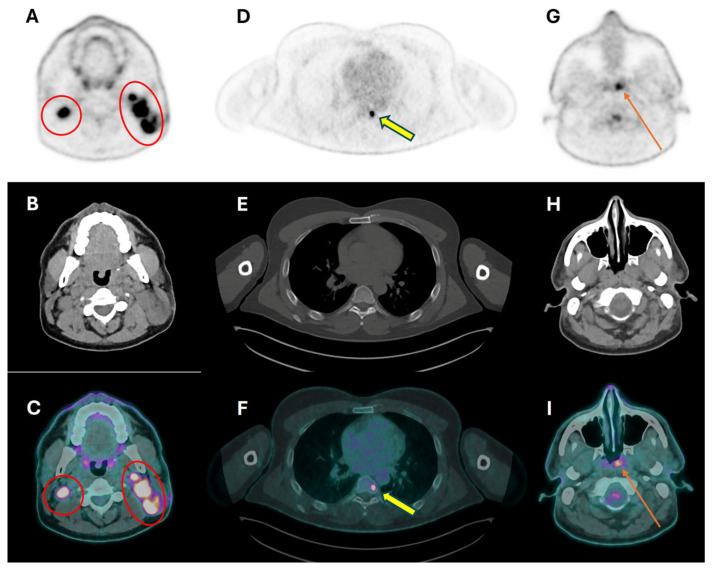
Axial PET, CT, and PET/CT images of a patient with cervical lymph node metastases of undifferentiated carcinoma, and no clinical evidence of primary lesion in the head–neck district. High ^18^F-FDG uptake is seen in enlarged cervical lymph nodes bilaterally (A,B), although more evident on the left side (red circles in (A,C)), and in the 7th dorsal vertebra (yellow arrow in (D,F)) without structural alterations at low-dose CT (E). PET/CT scan revealed the otherwise unknown primary nasopharyngeal tumor, on the left side (long arrow in (G,I)), not apparent on low-dose CT (H).
